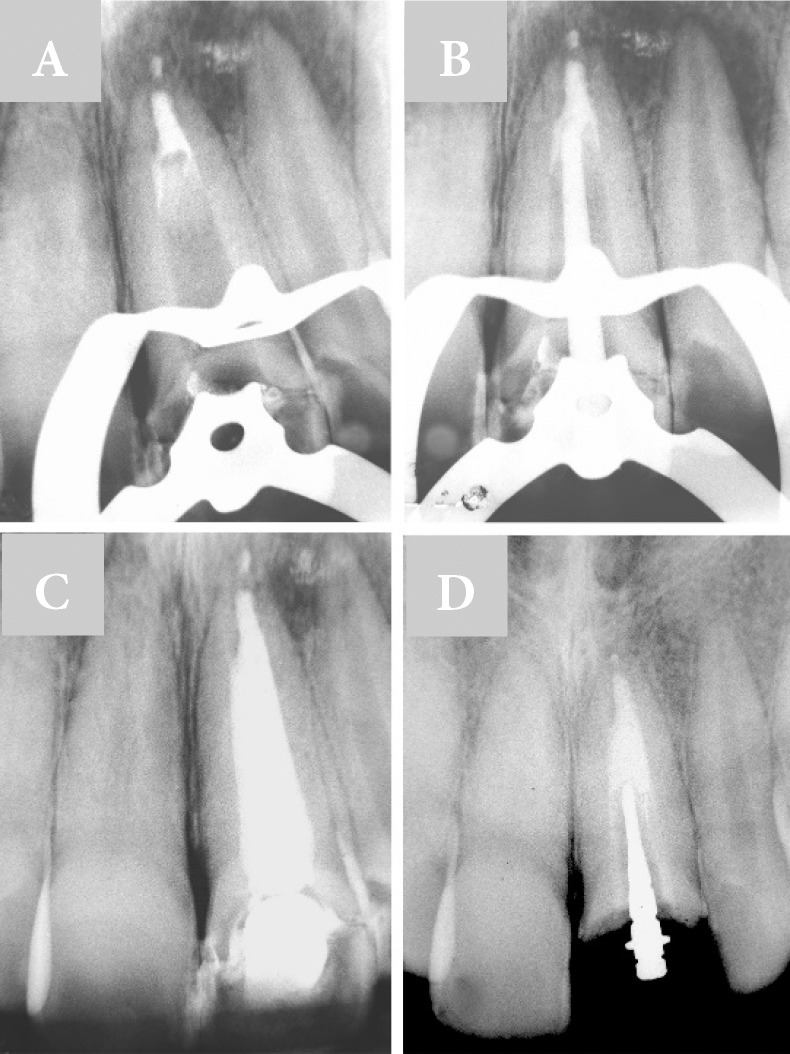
A) Lyophilized collagen sponge and MTA cement apical barrier; B) Apical barrier checking with gutta-percha point; C) Immediate root canal filling with calcium silicate-based sealer; D) Radiographic image after18 months
